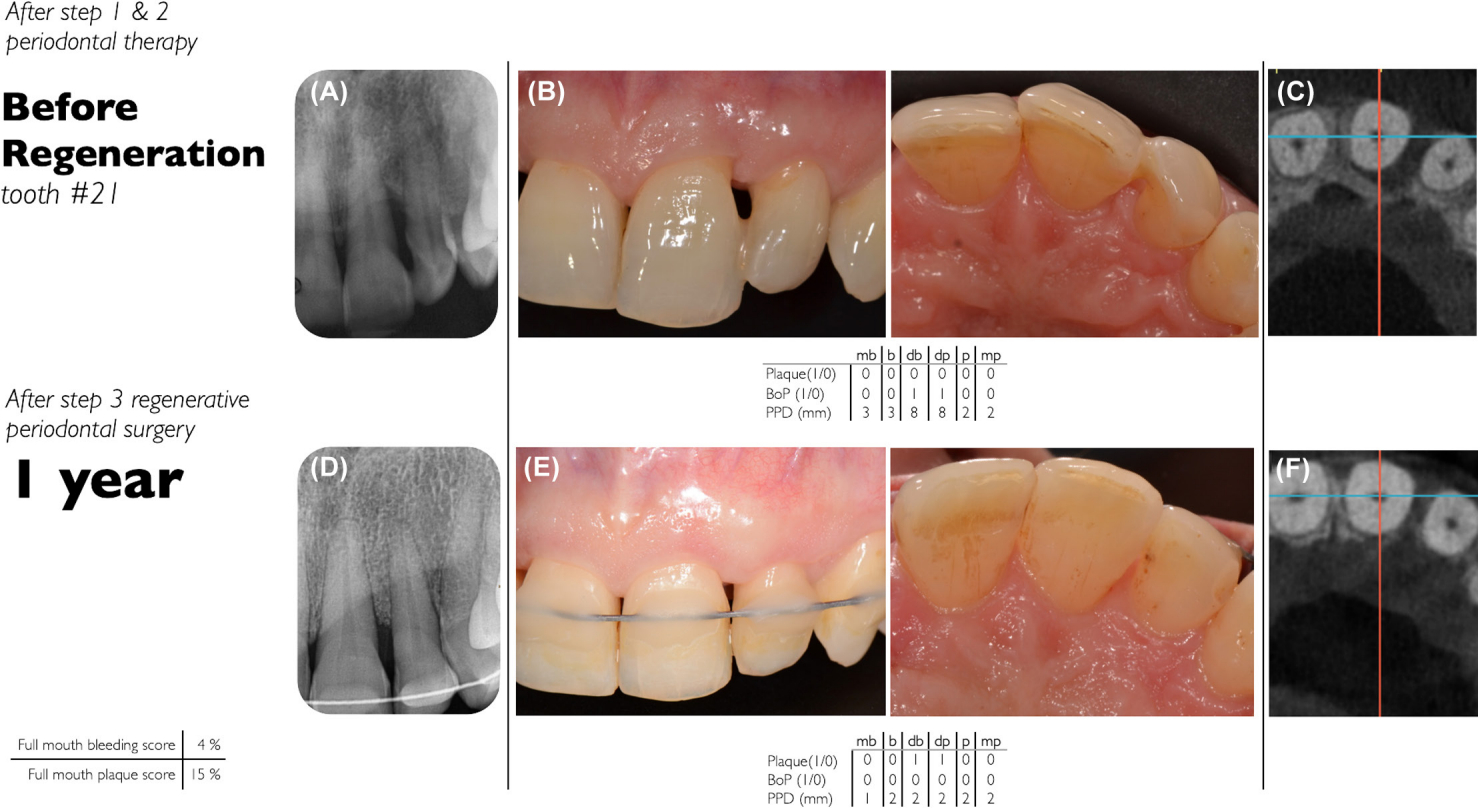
Radiographic and clinical situation of an upper left lateral incisor (#21) in a 45‐year‐old otherwise healthy patient after step 1 and 2 periodontal therapy and 1 year after OFD + Natural Bone Mineral + PRF. (A) Radiographic view with 2‐wall intrabony defect with bone loss ≥60%, (B) clinical attachment loss at the distal aspect 11mm of #21, mobility grade II, (C) CBCT with evidence of severe bone loss around tooth #21 extending to the mesio‐palatal aspect, (D) one‐year follow‐up of lesion with no evidence of radiographic pathologies, (E) clinical view with #21 still splinted with shallow probings, no recession and no bleeding on probing, (F) CBCT view with no more evidence of bone loss.
